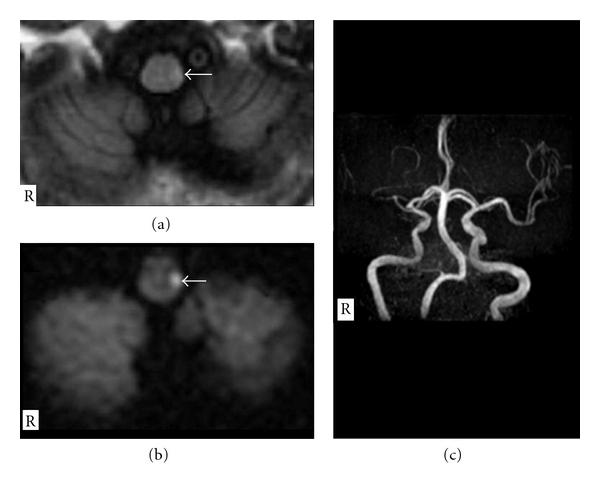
(a) Fluid-attenuated inversion recovery image and (b) diffusion-weighted image of cranial magnetic resonance imaging on the axial images demonstrated a small infarct lesion in the lower lateral medulla oblongata on the left side (arrow). (c) Cranial magnetic resonance angiography demonstrated an absence of flow of the left vertebral artery.
